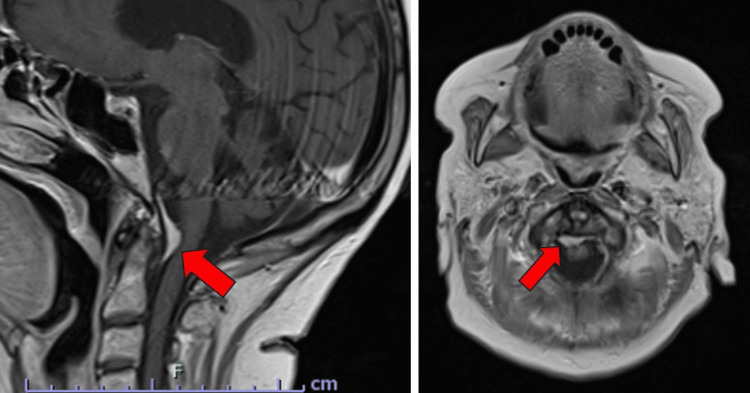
ZAP-X treatment planning MRI images of Patient 2. T1-weighted, contrast-enhanced MRI images of Patient 2, sagittal (A) and axial (B) sections, showing 1.3×0.7×2.6 cm meningioma impinging on the cervical spinal cord. Red arrows indicate cervical meningioma.
